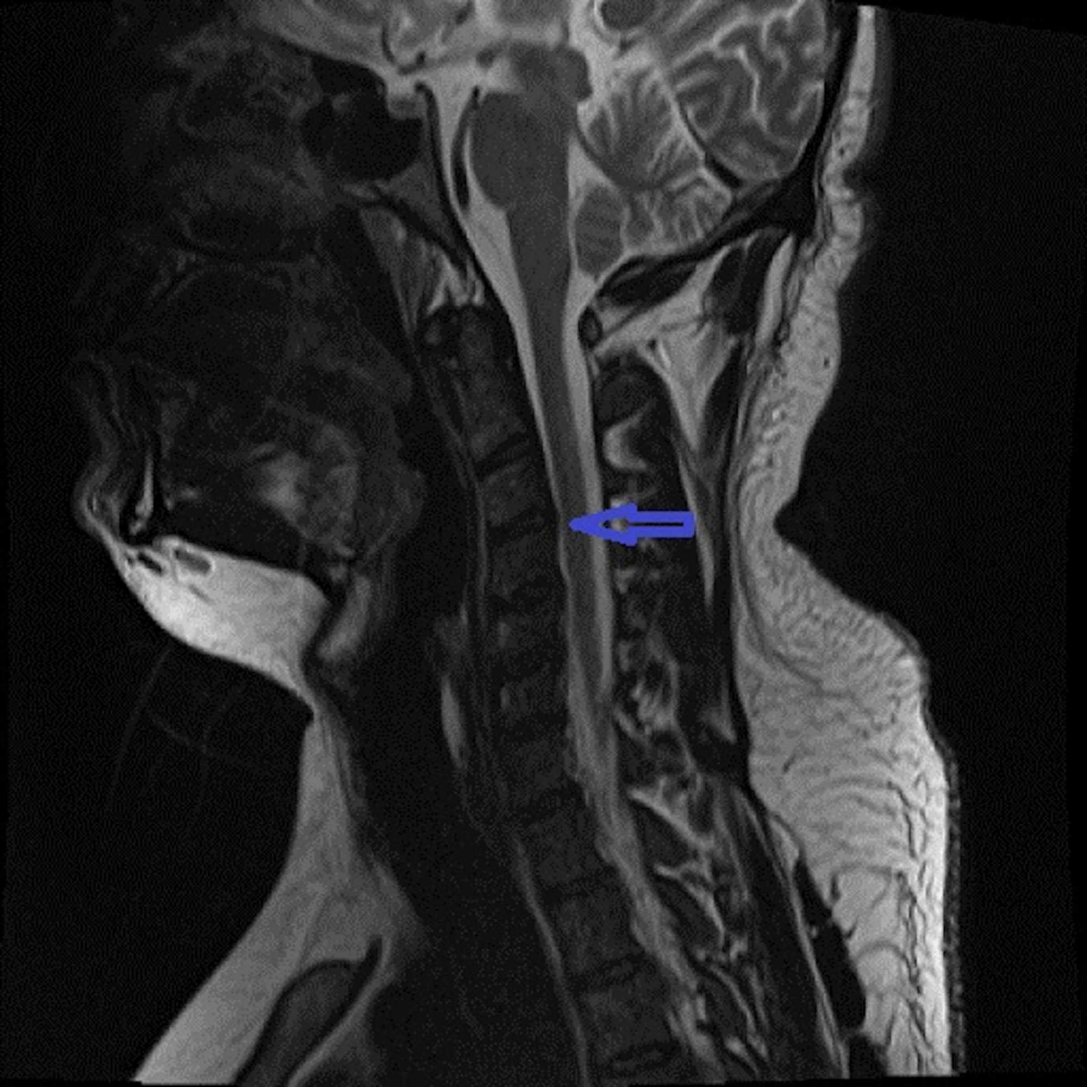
Magnetic Resonance Imaging (MRI) of the cervical spinal cord Sagittal T2- weighted MRI showed hyper-intense intra-medullary signal opposite C2-C3 through C4-C5 level (arrow).
